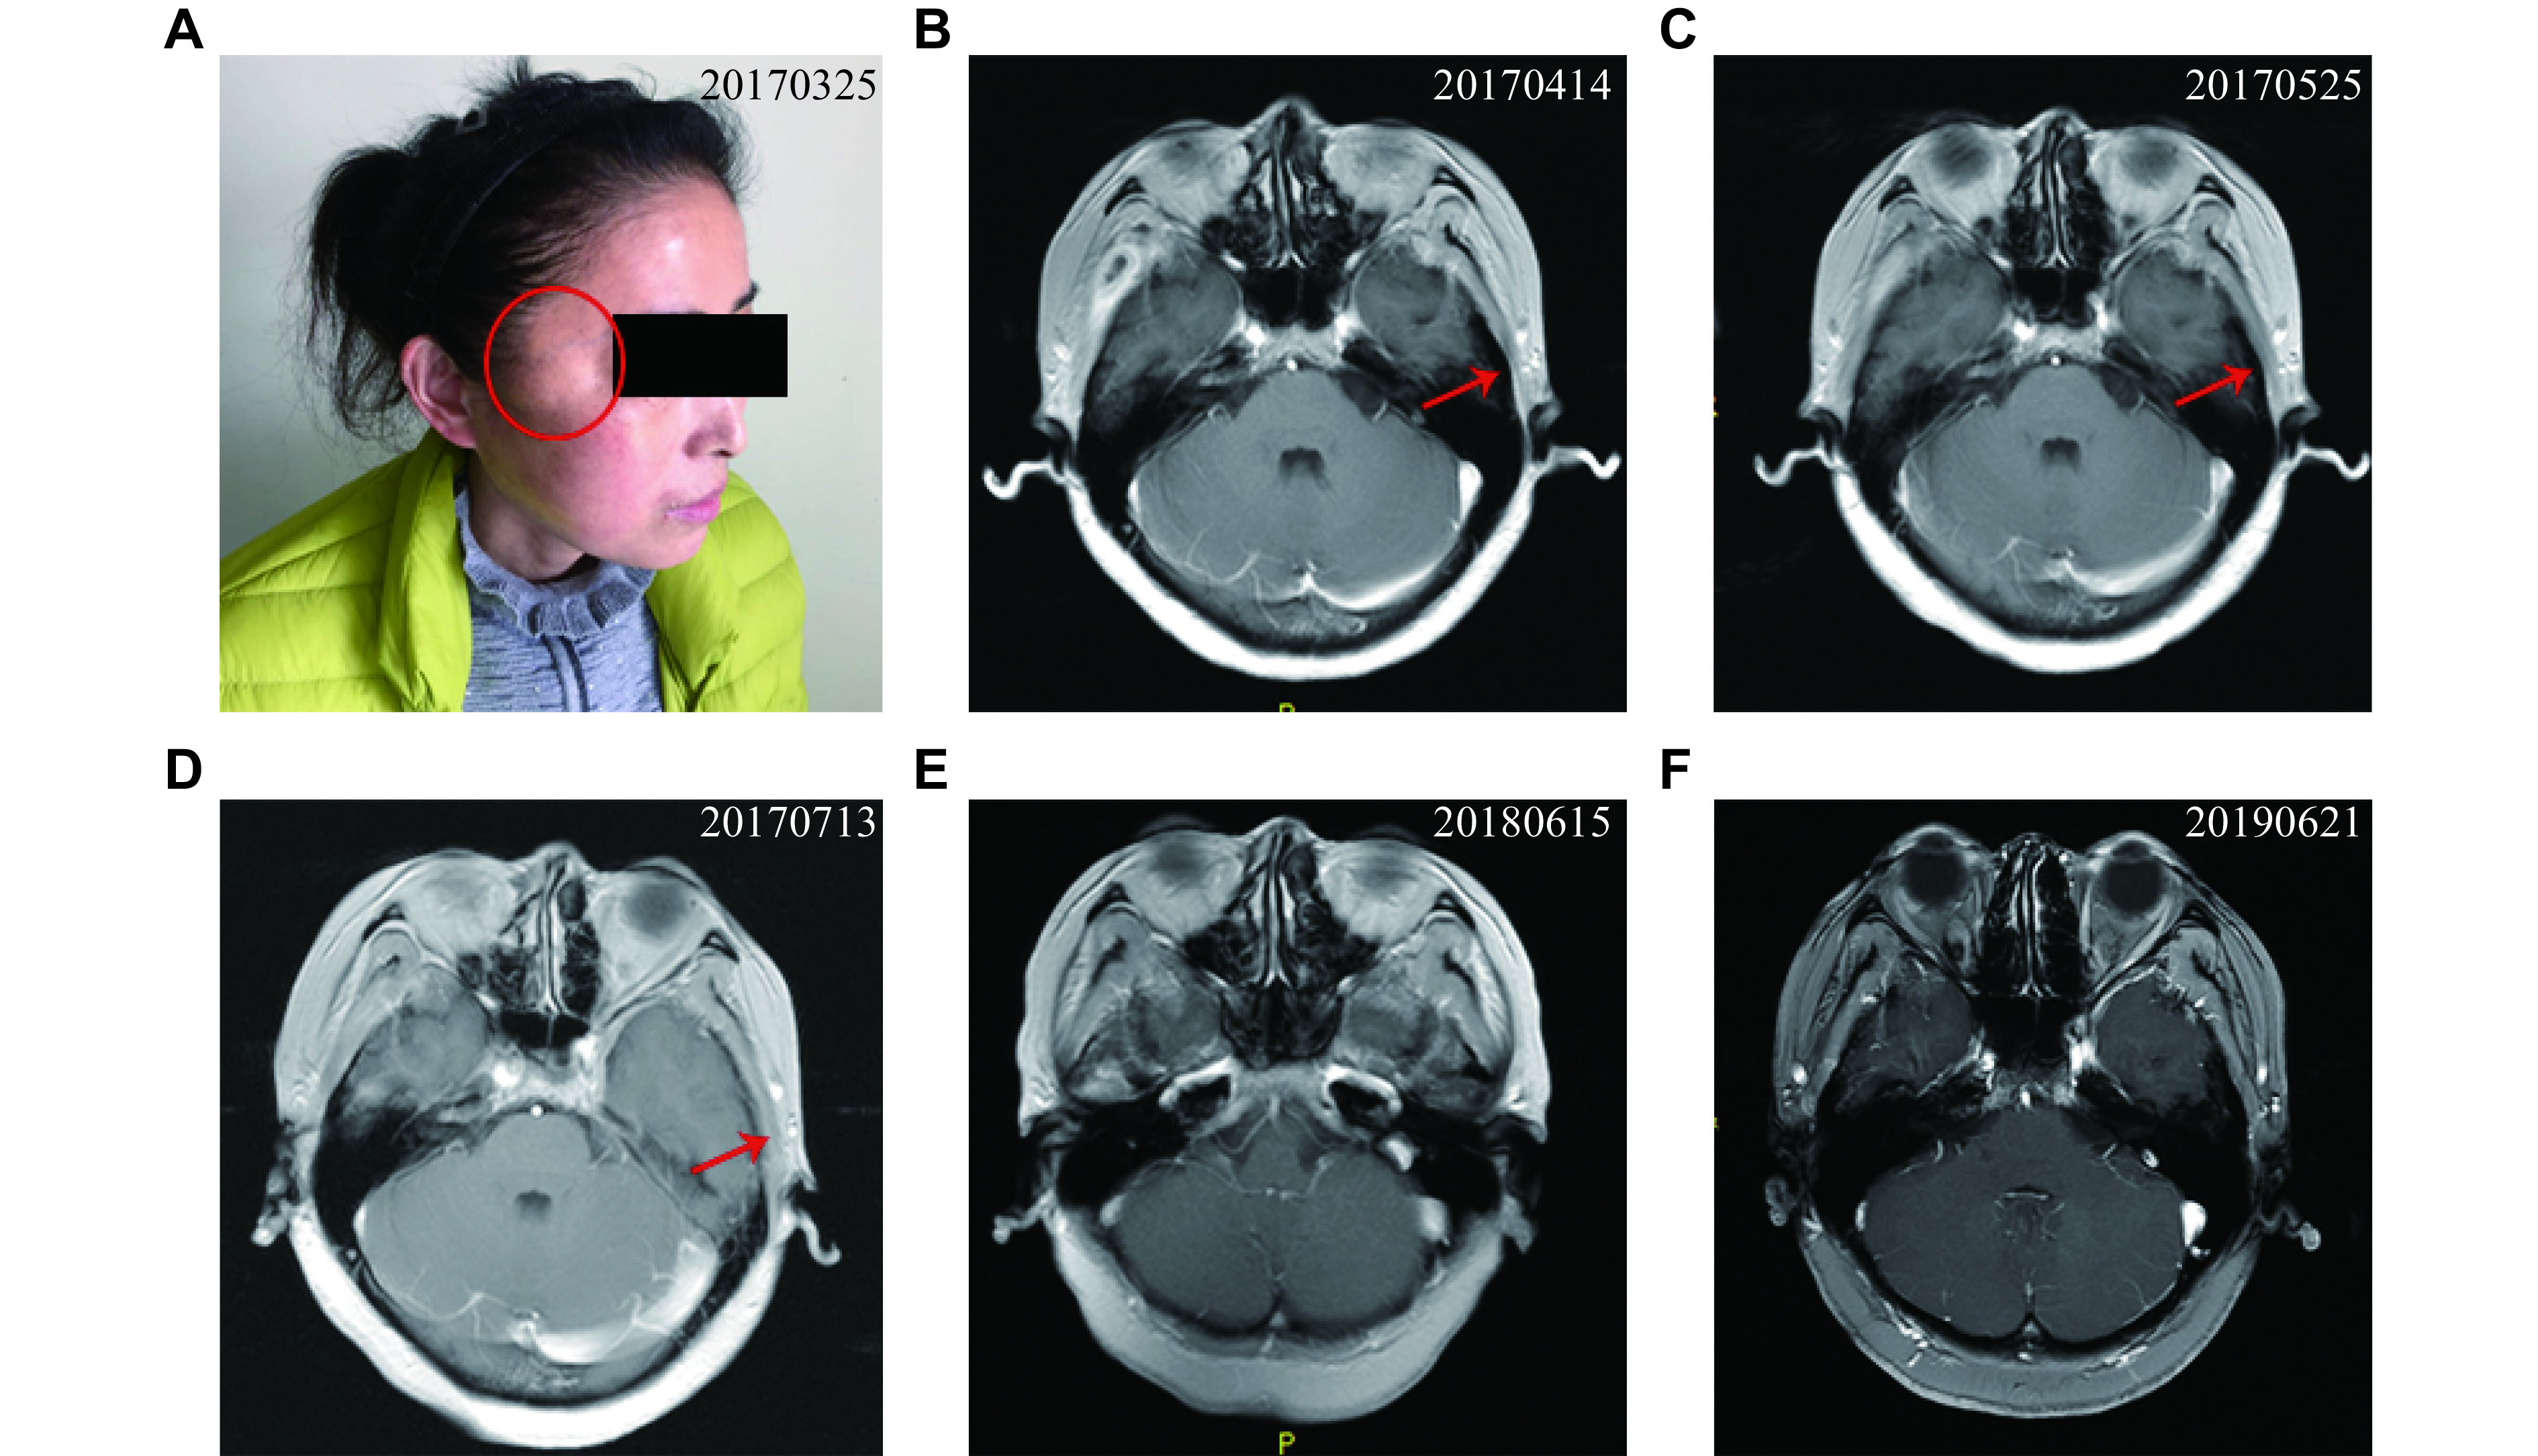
Magnetic resonance imaging (MRI) views revealed an oval lesion located within the right temporal muscle.
